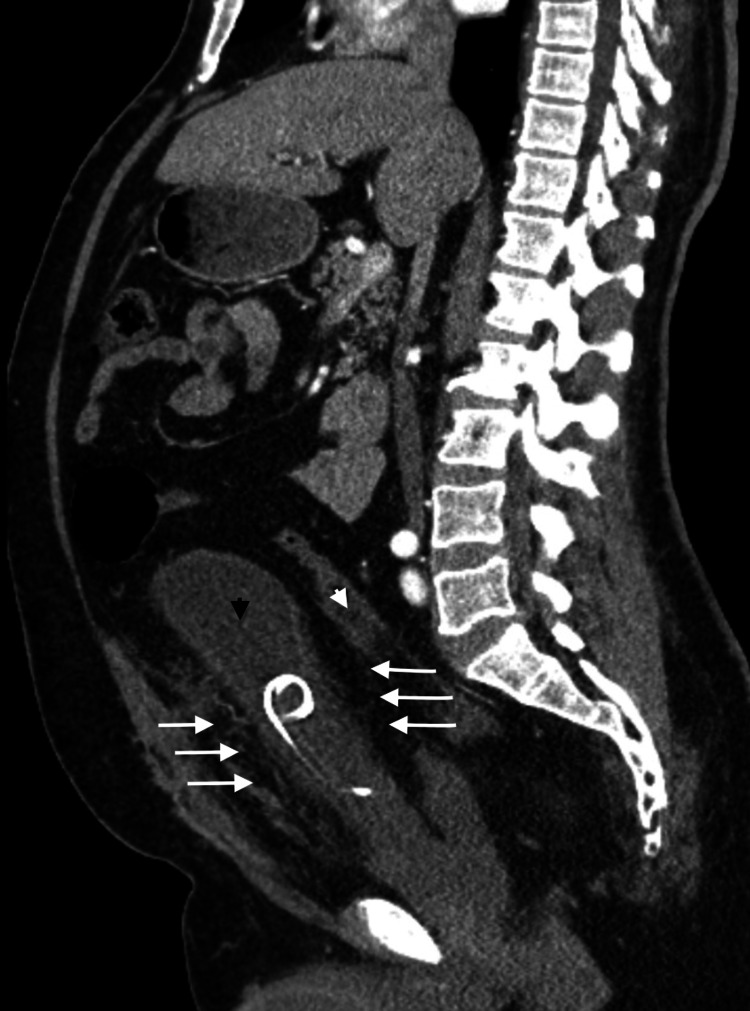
Pre-operative CT (sagittal view) Pear-shaped elongated urinary bladder (black arrowhead) and compressed rectum (white arrowhead) secondary to extensive pelvic lipomatosis (white arrows).
